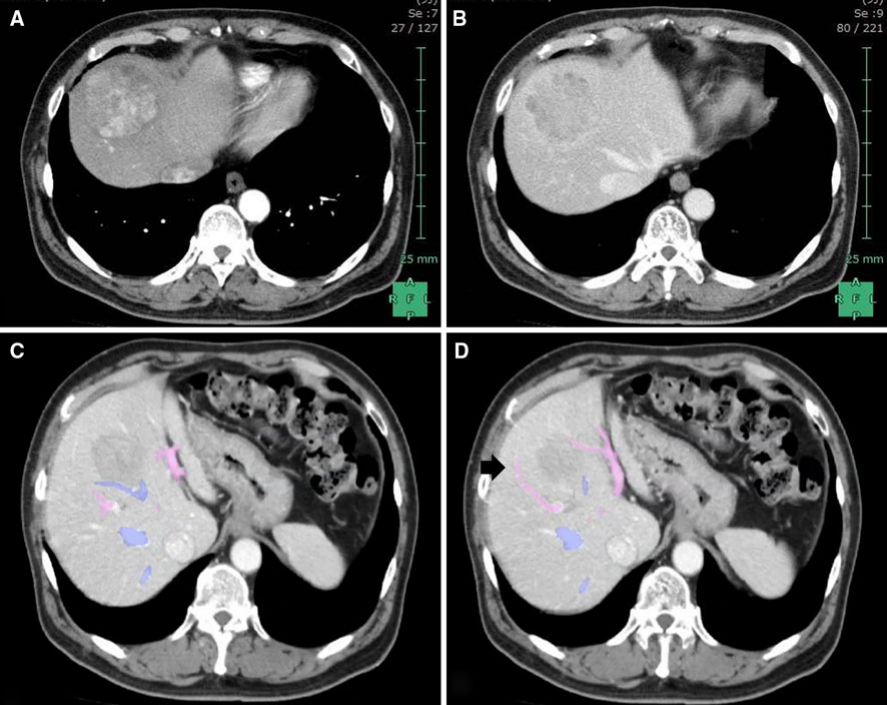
Enhanced abdominal computed tomographic image showing A early arterial phase of HCC centrally located in the liver and B, C compression of the MHV. D Ventral branch of the right anterior portal trunk is pointed to by the *arrow*. *Pink* portal vein, *blue* hepatic vein (Color figure online)
